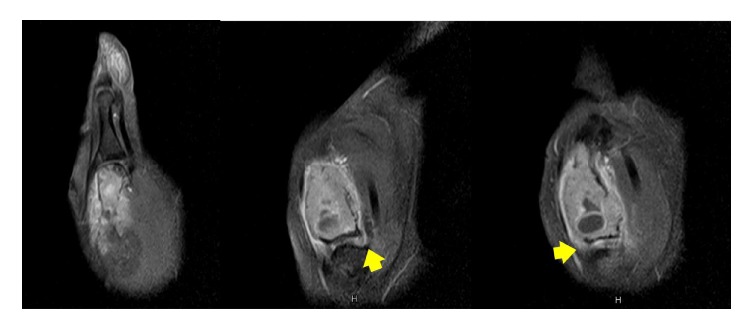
MRI showing the extension of the tumor into the surrounding soft tissue and around the first carpometacarpal joint (yellow arrow).
